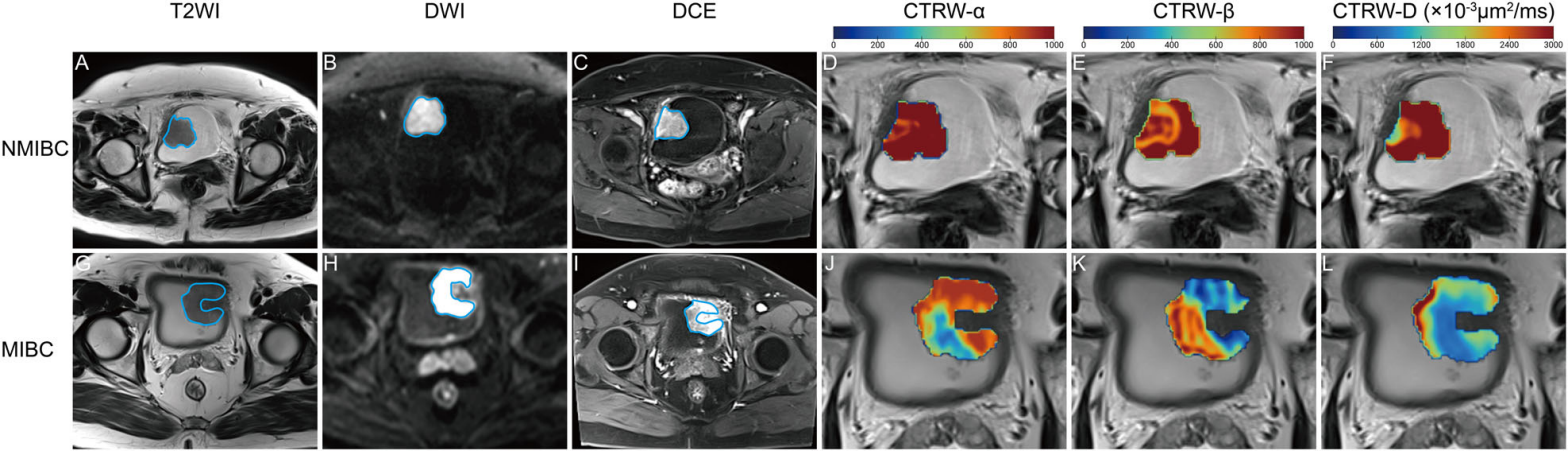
T2-weighted (T2WI), conventional diffusion-weighted (DWI), and dynamic contrast-enhanced (DCE) images, and CTRW metric maps from (A–F) a 69-year-old male with non-muscle-invasive bladder cancer (NMIBC) and (G–L) a 60-year-old male with muscle-invasive bladder cancer (MIBC). A, G T2WI, (B, H) DWI, and (C, I) DCE, along with (D, J) CTRW-α, (E, K) CTRW-β, and (F, L) CTRW-D metric maps integrated into the corresponding T2WI within the tumors. In both patients, the Vesical Imaging Reporting and Data System assigned a score of 3. Additionally, all metric maps indicated that the lesion in the MIBC appeared cooler and exhibited more variability in color, suggesting a higher degree of diffusion limitation and greater heterogeneity, both temporally and spatially, within the lesion
